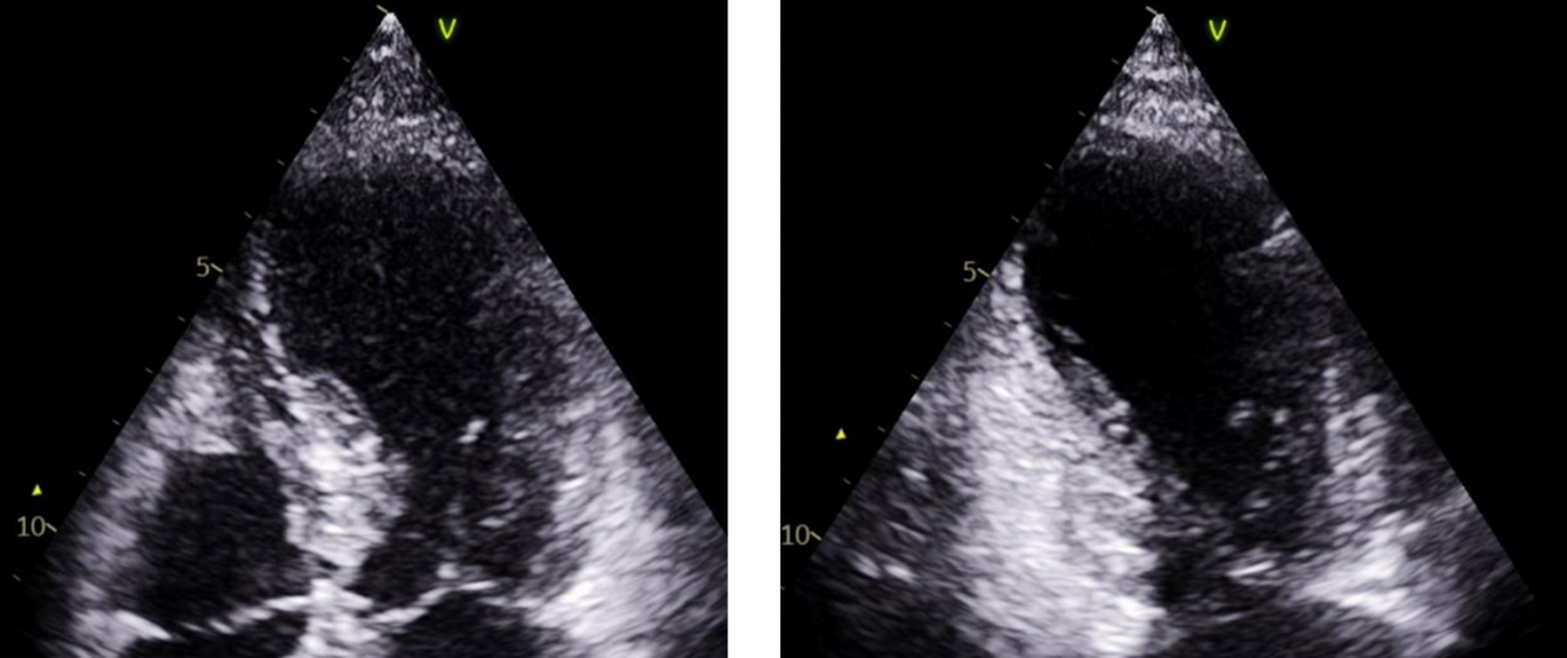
Transthoracic echocardiography apical 4‐chamber (A) and 2‐chamber (B) views at end‐systole showing apical akinesia.
